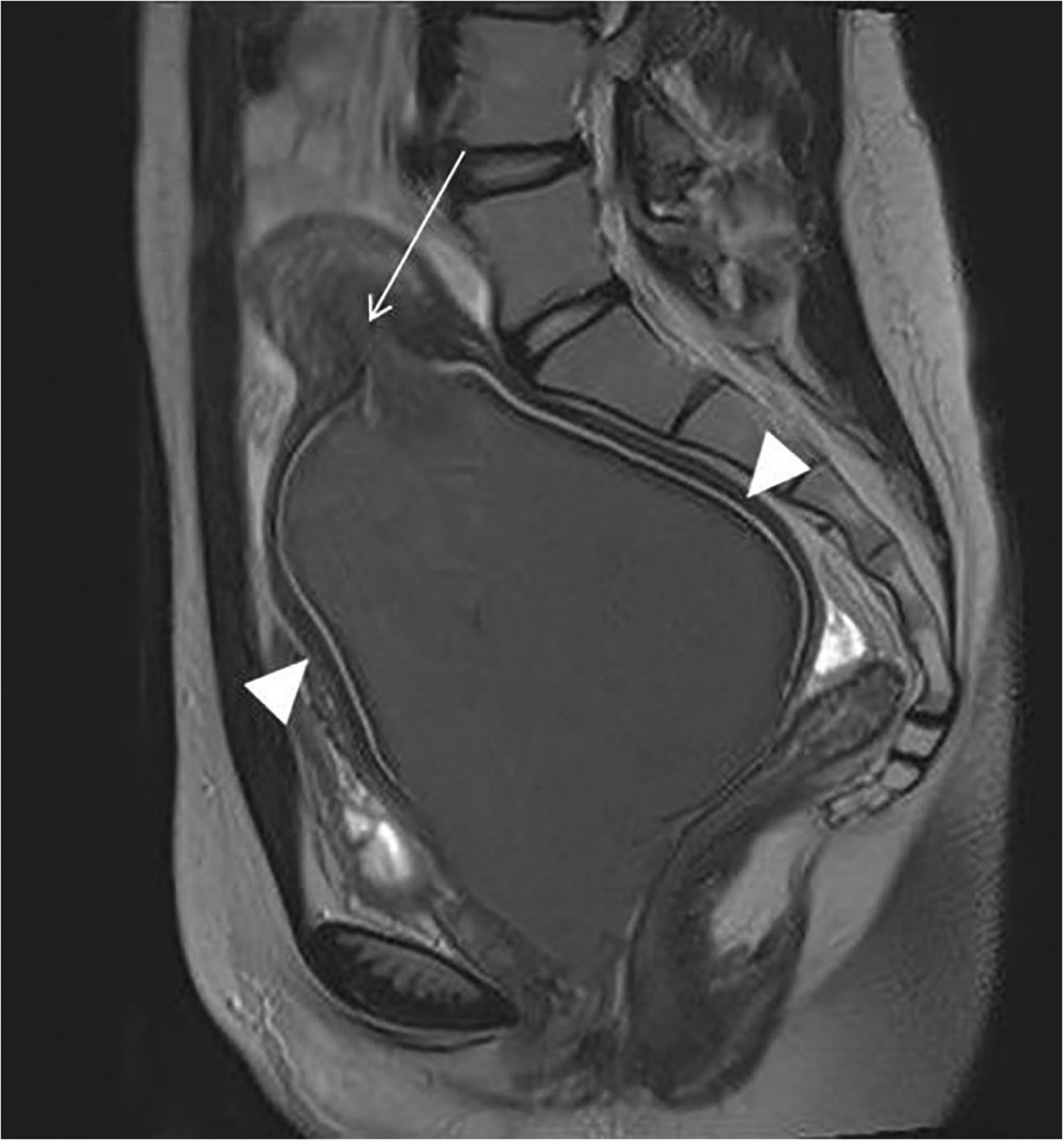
T2-weighted sagittal image of the distended cervix. The uterine cavity (arrow) is only slightly distended. The cervical stroma (arrow heads) is considerably stretched
